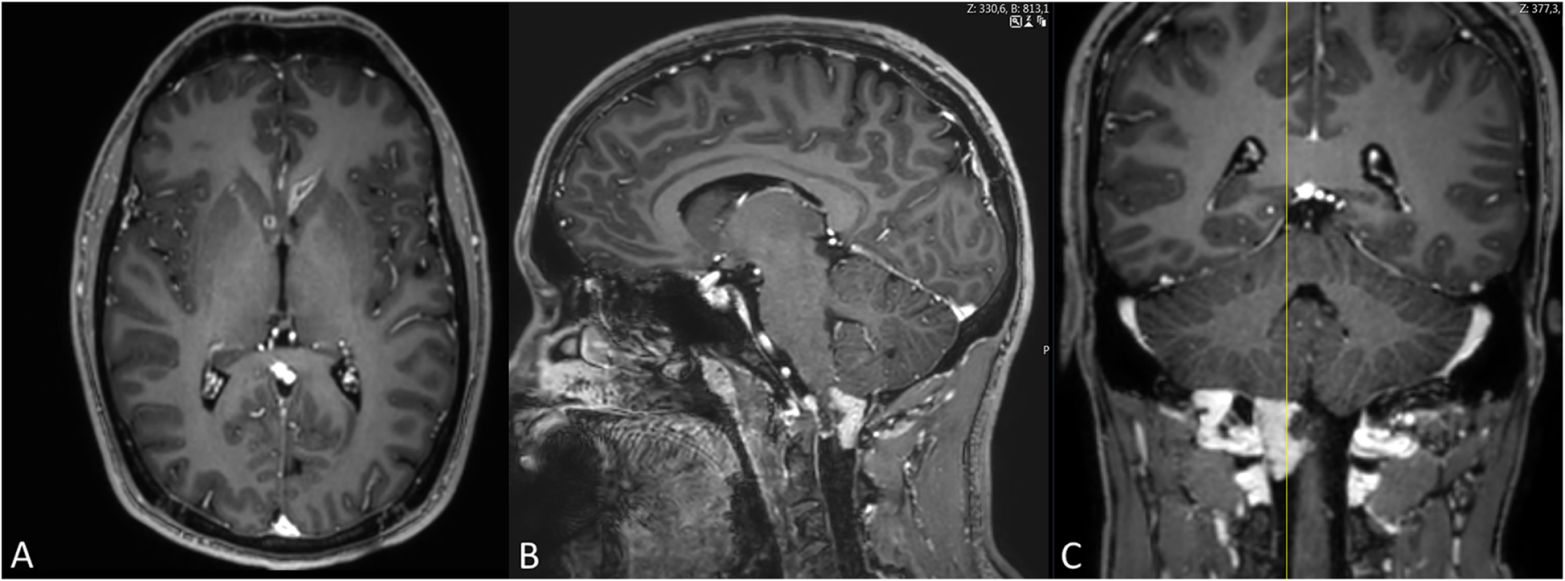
Cerebral contrast-enhanced T1-weighted MR scans present multiple metastatic tumor lesions. A contrast-enhanced axial T1-weighted MRI demonstrates lesions at the left lateral ventricle and the right caudate nucleus. B, C Contrast-enhanced sagittal (B) and coronary (C) T1-weighted MRI shows a large metastatic lesion at the right craniocervical transition
